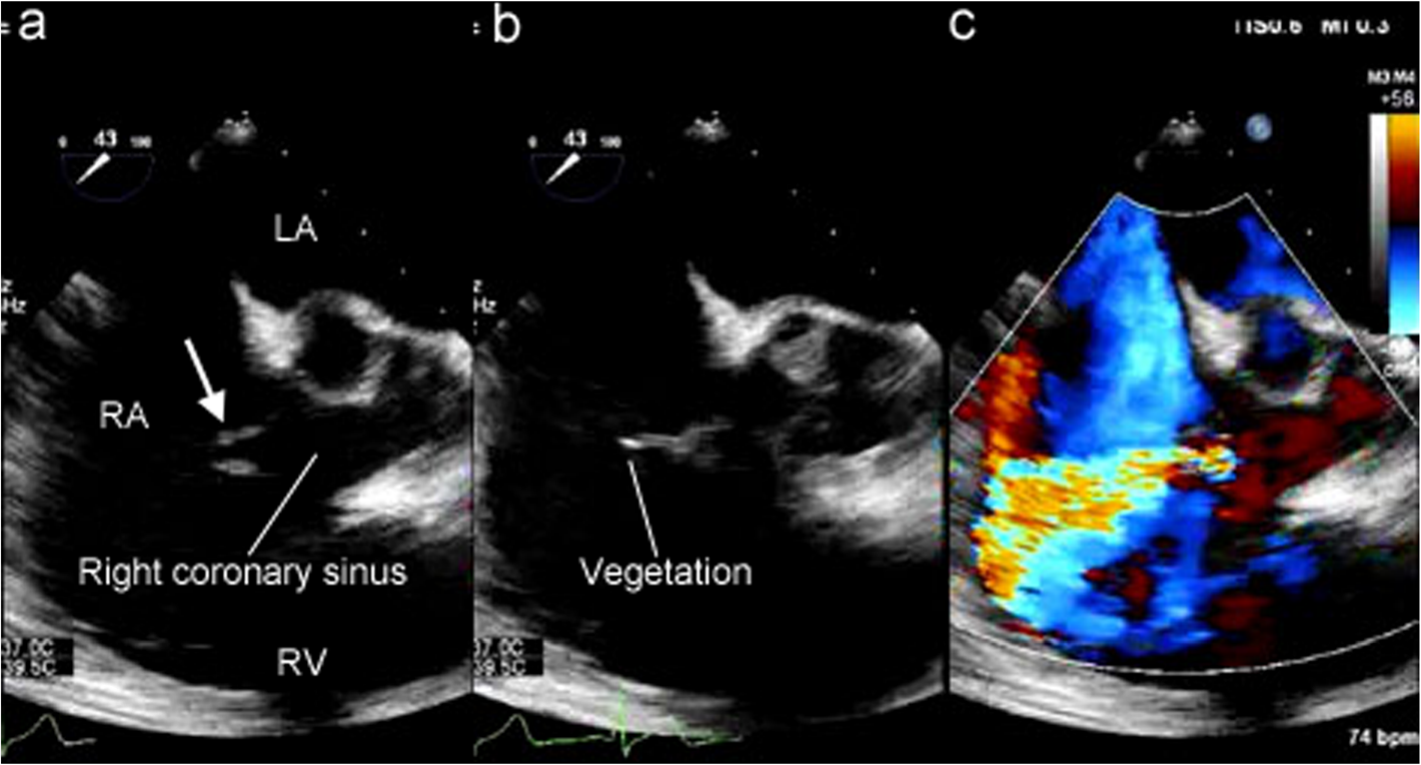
a Preoperative transesophageal echocardiography showing a windsock-like fistula tract originating in the right coronary sinus protruding into the right atrium (*arrow*). b String-shaped vegetation was attached to the tip of the windsock-like fistula. c An L-R shunt via the fistula tract was seen. *LA* left atrium, *RA* right atrium, *RV* right ventricle
